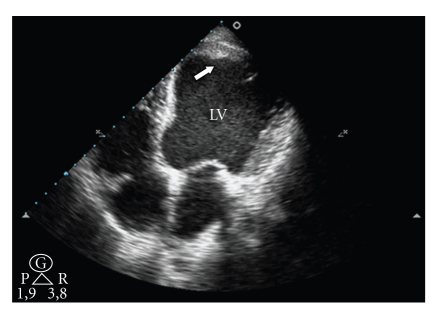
Transthoracic echocardiogram image showing thrombus sessile (white arrow) on left ventricular apex (LV).
